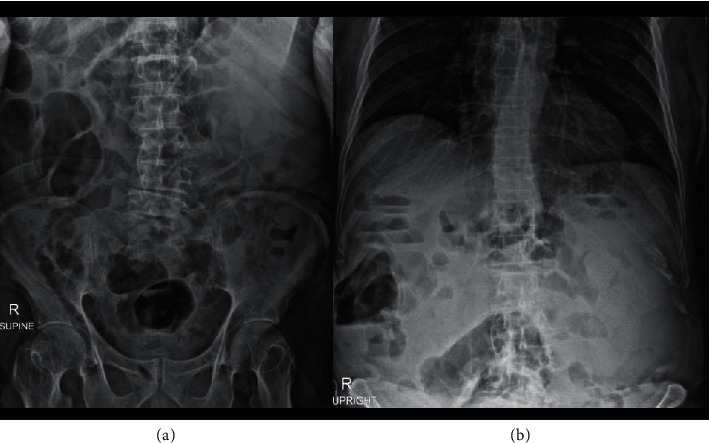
Supine (a) and upright (b) abdominal radiographs revealed a large well-defined soft tissue opacity at the left upper quadrant of abdomen, deviating left-sided colon medially. No signs of bowel obstruction were noted.
